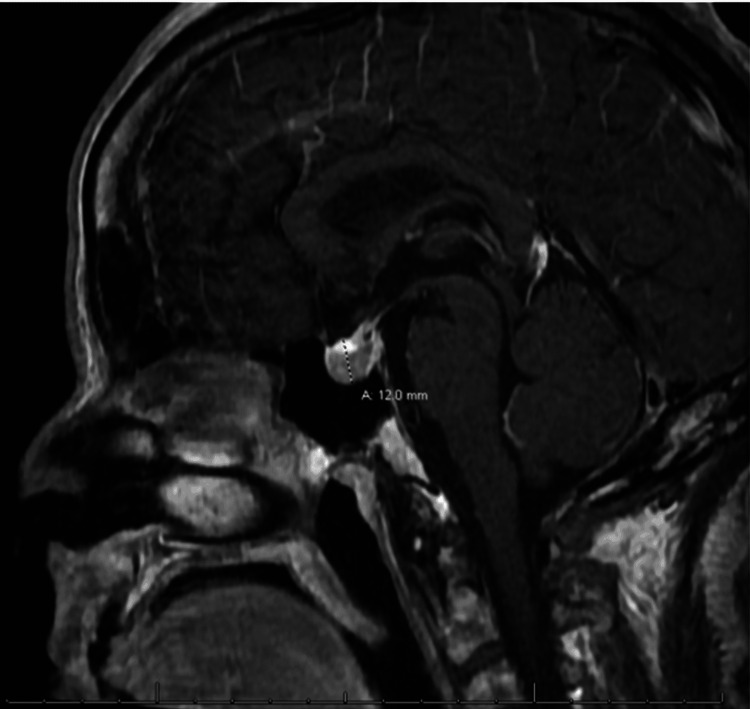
MRI brain sagittal view of 12 mm pituitary adenoma. Post-operative MRI showed complete resolution of pituitary adenoma.
